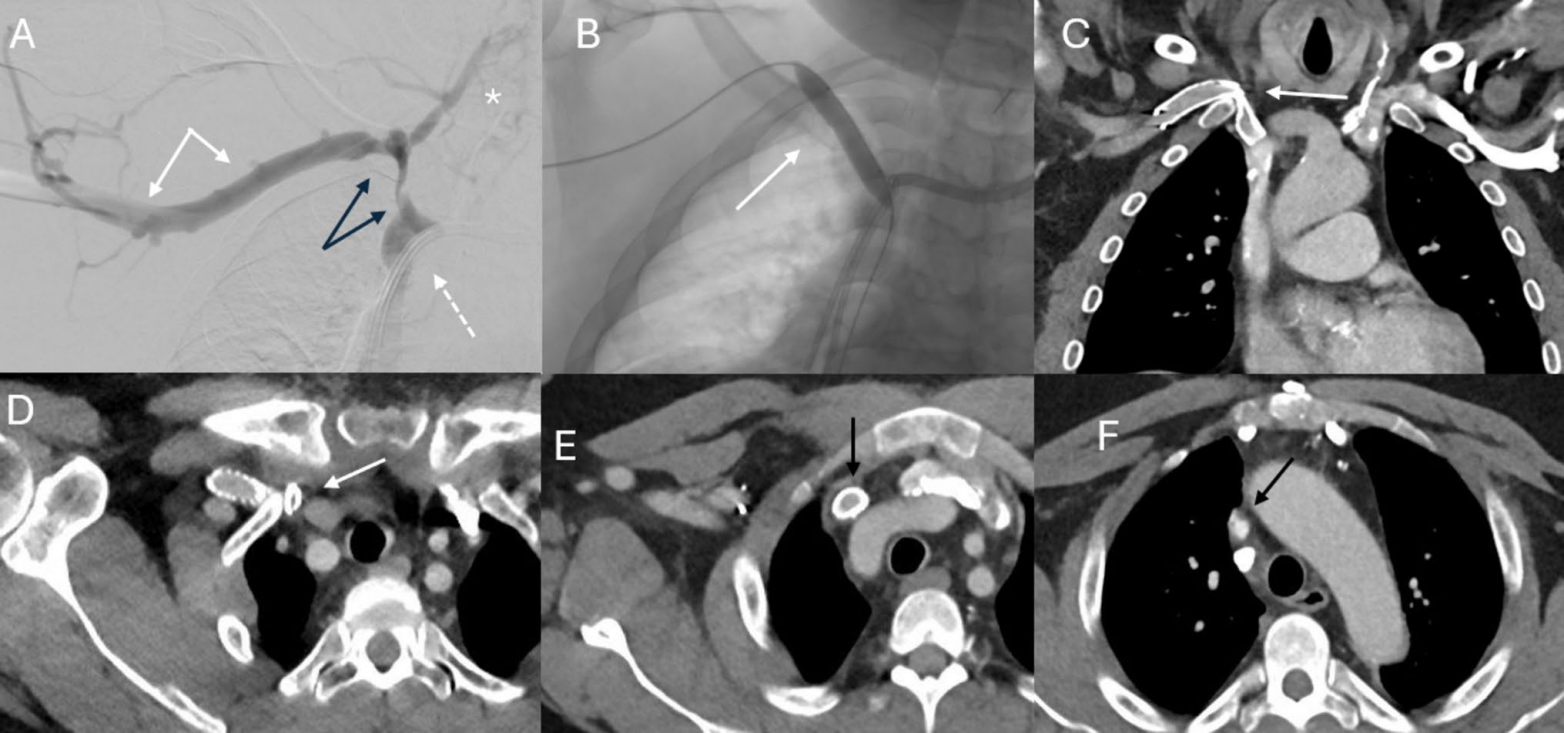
A 52 year-old male with right upper extremity brachiocephalic fistula for hemodialysis who presented with arm swelling and poor flow rates during dialysis. The patient had a history of repeated endovascular interventions. Digital subtraction fistulogram demonstrated patent brachial and axillary veins (white arrows) as well as severe stenotic disease involving the proximal right subclavian vein and right innominate vein (A). Collateral venous vasculature visualized and marked with asterisk (A). Due to dysfunction of the fistula, a left internal jugular vein tunneled dialysis catheter was placed (white dashed arrow in A). Subsequent balloon angioplasty of the long segment severe stenosis was performed using 12 mm compliant balloon (white arrow in B) without stent placement at the time of the procedure. Subsequently, a stent graft was placed at the outside hospital and follow-up CT angiography was performed due to persistently decreased flow rates during hemodialysis in anticipation of surgical vascular access revision. Stent compression with acute kink at the thoracic inlet (white arrows) as seen on coronal reconstruction image (C) and axial image (D). Caudal portion of the stented right innominate vein is patent (E). Mild to moderate stenotic disease of the cranial portion of the superior vena cava is noted (black arrow) on axial slice of the CT angiography study (F)
